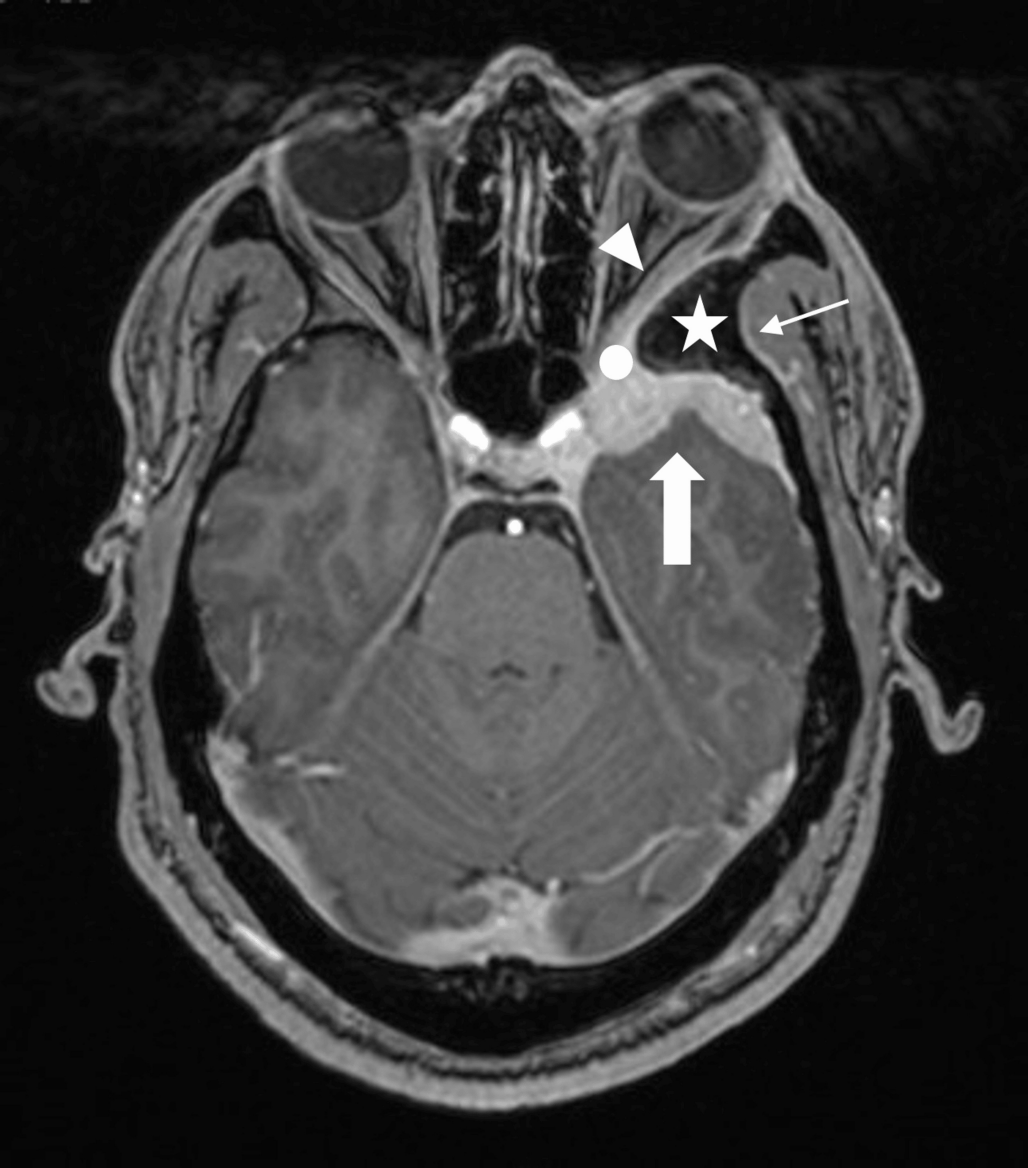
Preoperative axial T1-weighted brain MRI with gadolinium contrast. Enhancing lesion centered on the left greater wing of the sphenoid bone (white star), with associated meningeal thickening at the temporal apex (thick arrow), involvement of the orbital canal (white circle), extension into the orbit (arrowhead), and anterior portion of the cavernous sinus. Periosteal enhancement is also noted in the left temporal fossa (thin arrow).
